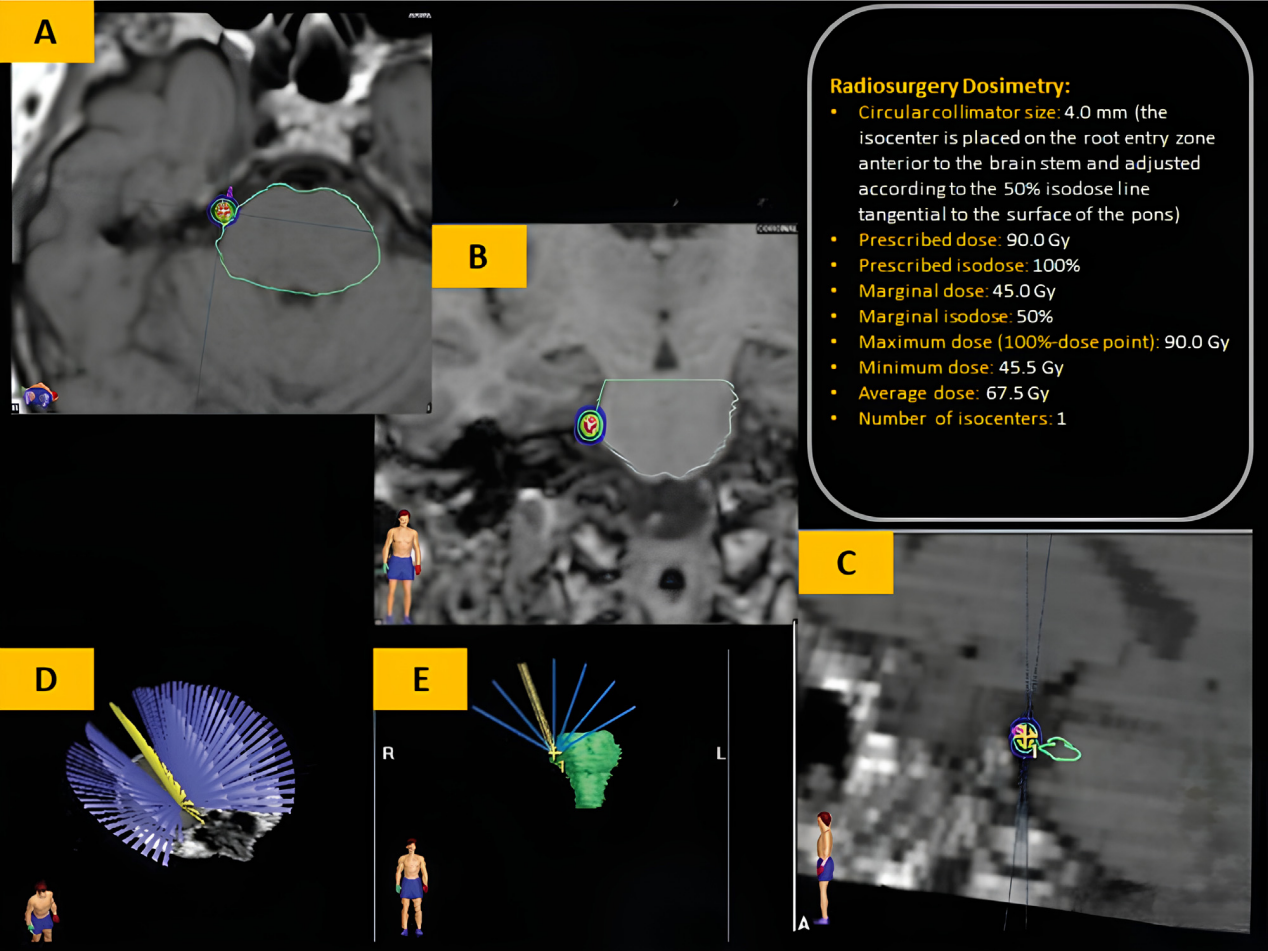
SRS Treatment Plan. Axial (A), coronal (B), and sagittal (C) T1-weighted images at the level of the trigeminal nerve. The SRS isocenter (*multicolored outline*) is placed on the trigeminal nerve root, 2-4 mm anterior to the point where the nerve emerges from the pons (*green outline*). The isocenter is adjusted according to the 50% isodose line, which is tangential to the surface of the pons (D and E).
